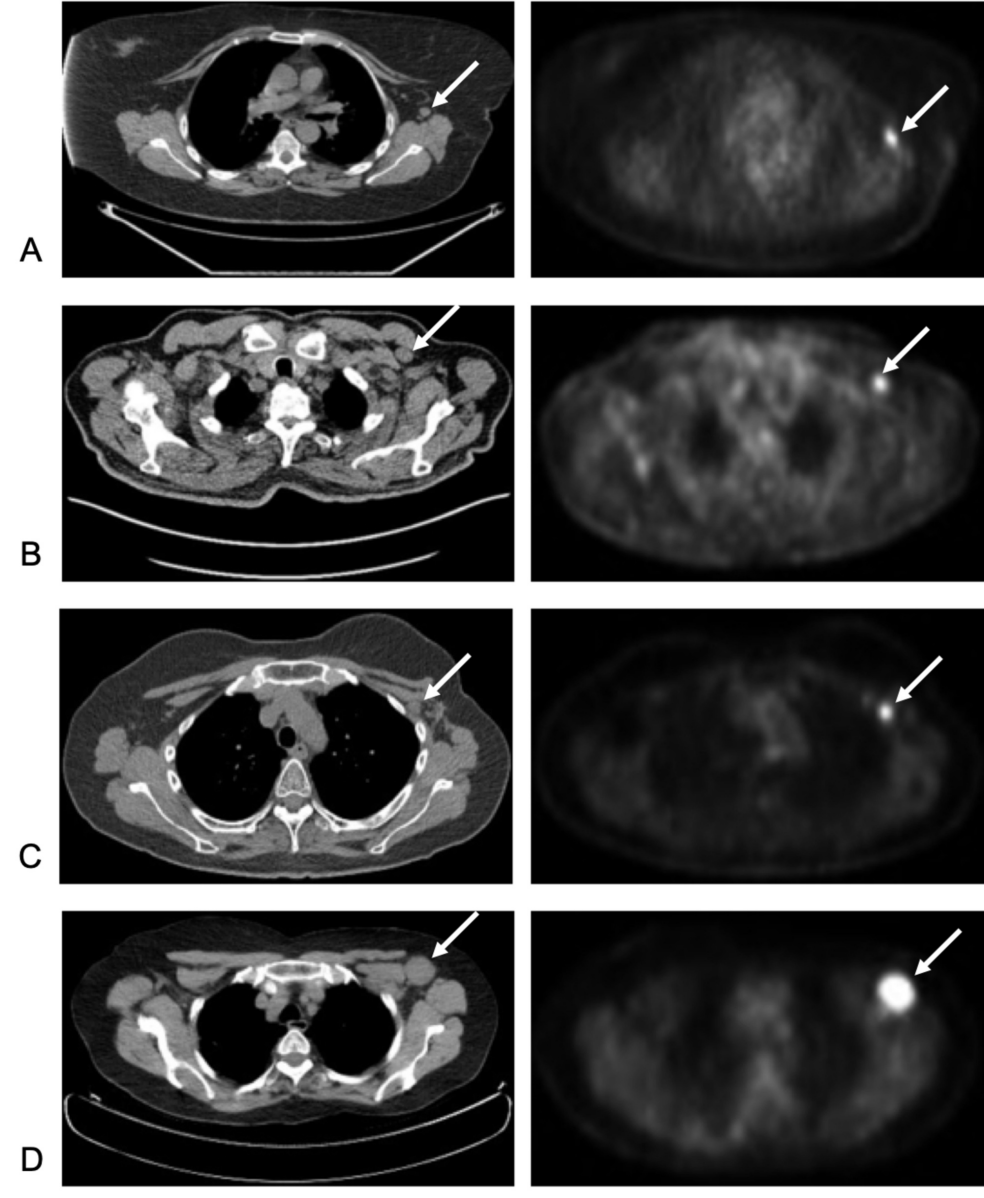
PET/CT images of A) case 1; B) case 2; C) case 3; and D) case 4. Arrows indicate the position of hypermetabolic lymphatic nodes.
